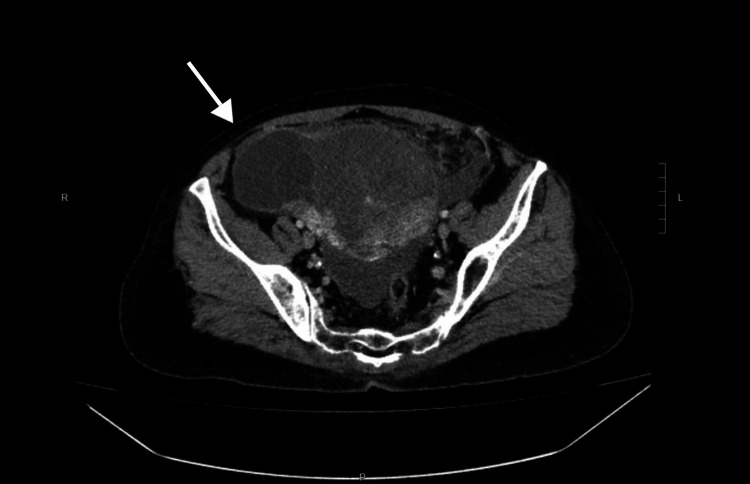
The CT scan showing a 15-cm pelvic mass.
